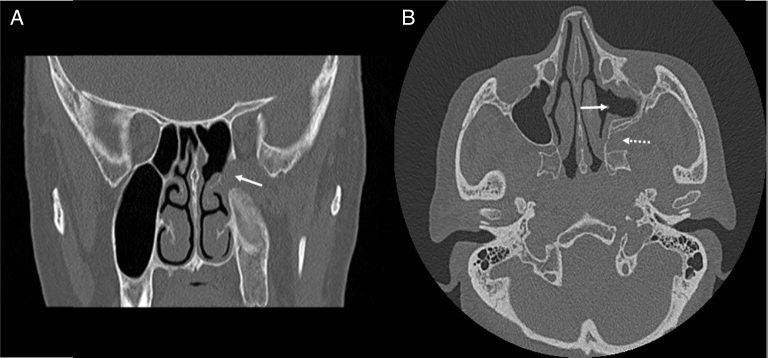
Postoperative CT images. (A) Coronal CT scan. There is no tumor recurrence in the pterygopalatine fossa (arrow indicates pterygopalatine fossa). (B) Axial CT scan. Left maxillary sinus and pterygopalatine fossa are seen normal (arrow indicates wide meatal antrostomy and dashed arrow indicates pterygopalatine fossa).
